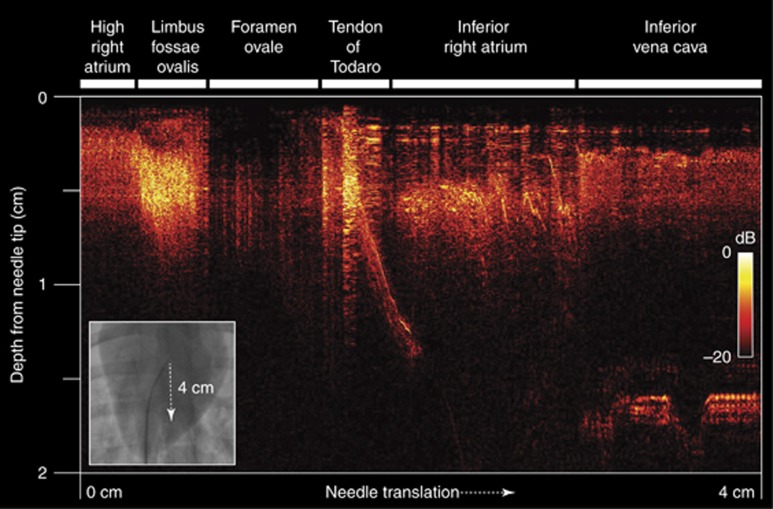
Two-dimensional all-optical ultrasound imaging (B-Mode) acquired during the manual translation of the needle tip across a distance of 4 cm. As the needle tip progressed from the high right atrium to the inferior vena cava, the thin foramen ovale manifested as a hypoechoic region between the thick limbus fossae ovalis and the tendon of Todaro (with a diagonal artifact from the ICE catheter and sheath). X-ray fluoroscopic imaging was acquired concurrently (inset).
